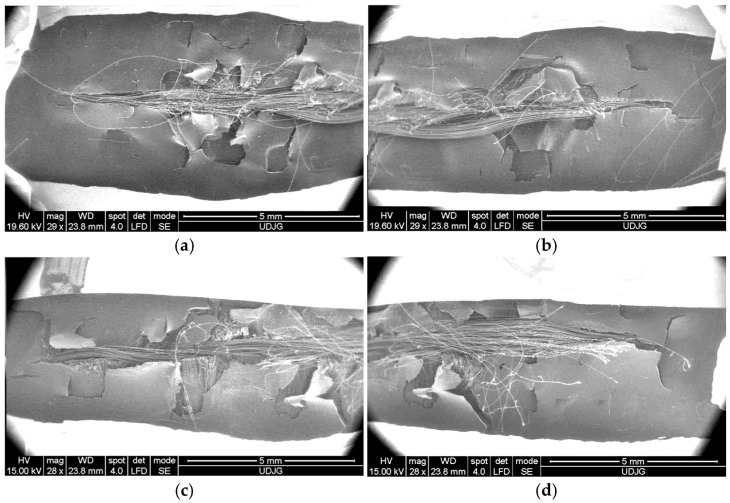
SEM images of the knife cut in the panel made of 16 layers of Twaron^®^ CT 736 CMP (thickness of 8.44 mm), after a test with a strike energy of 24 J: (a) left side of the cut on the impacted face; (b) right side of the cut on the impacted face; (c) left side of the cut on the back face of the last layer; (d) right side of the cut on the back face of the last layer.
